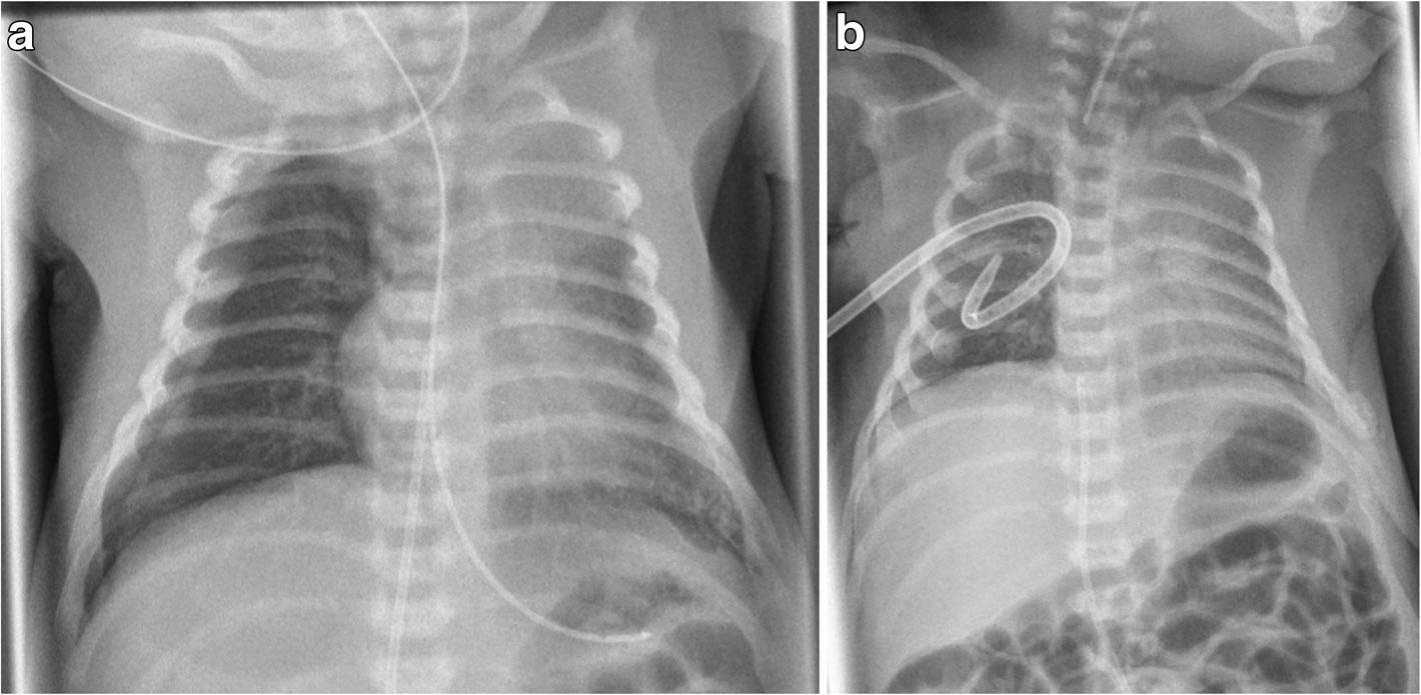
Chest X-rays on the first day of life. a spontaneous right-sided pneumothorax b) after intubation and chest tube insertion
